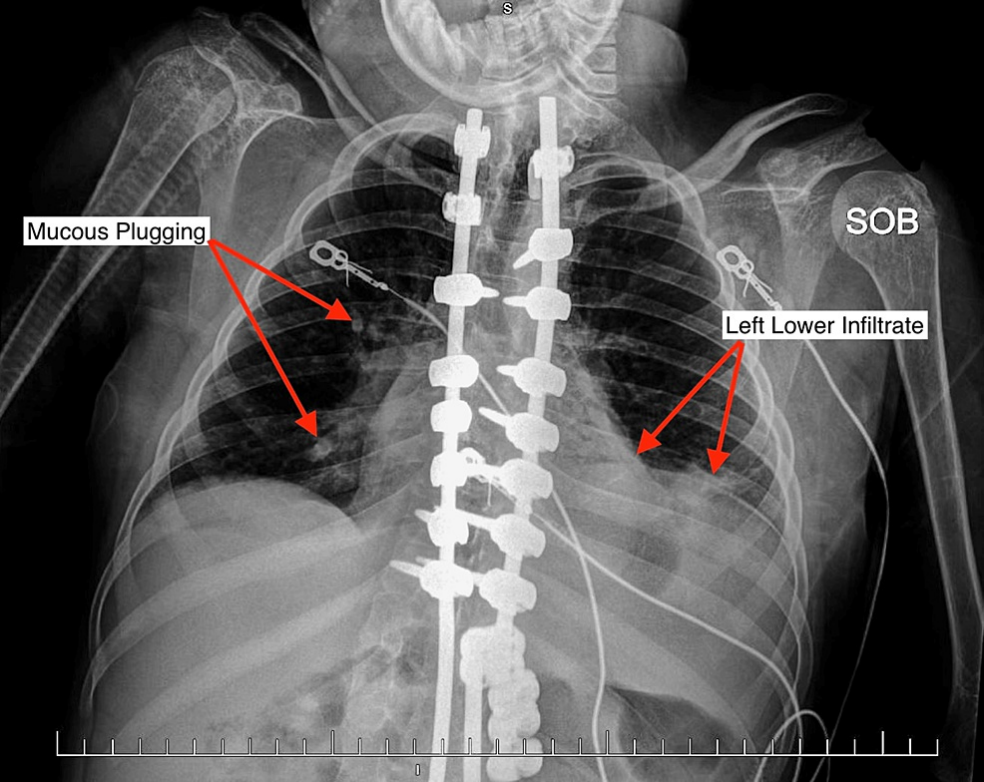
This is the patients first chest radiography, taken in the ED. The findings, labeled on the radiograph, were remarkable for a left lower lobe infiltrate, mild left-sided pleural effusion, and moderate mucous plugging.
